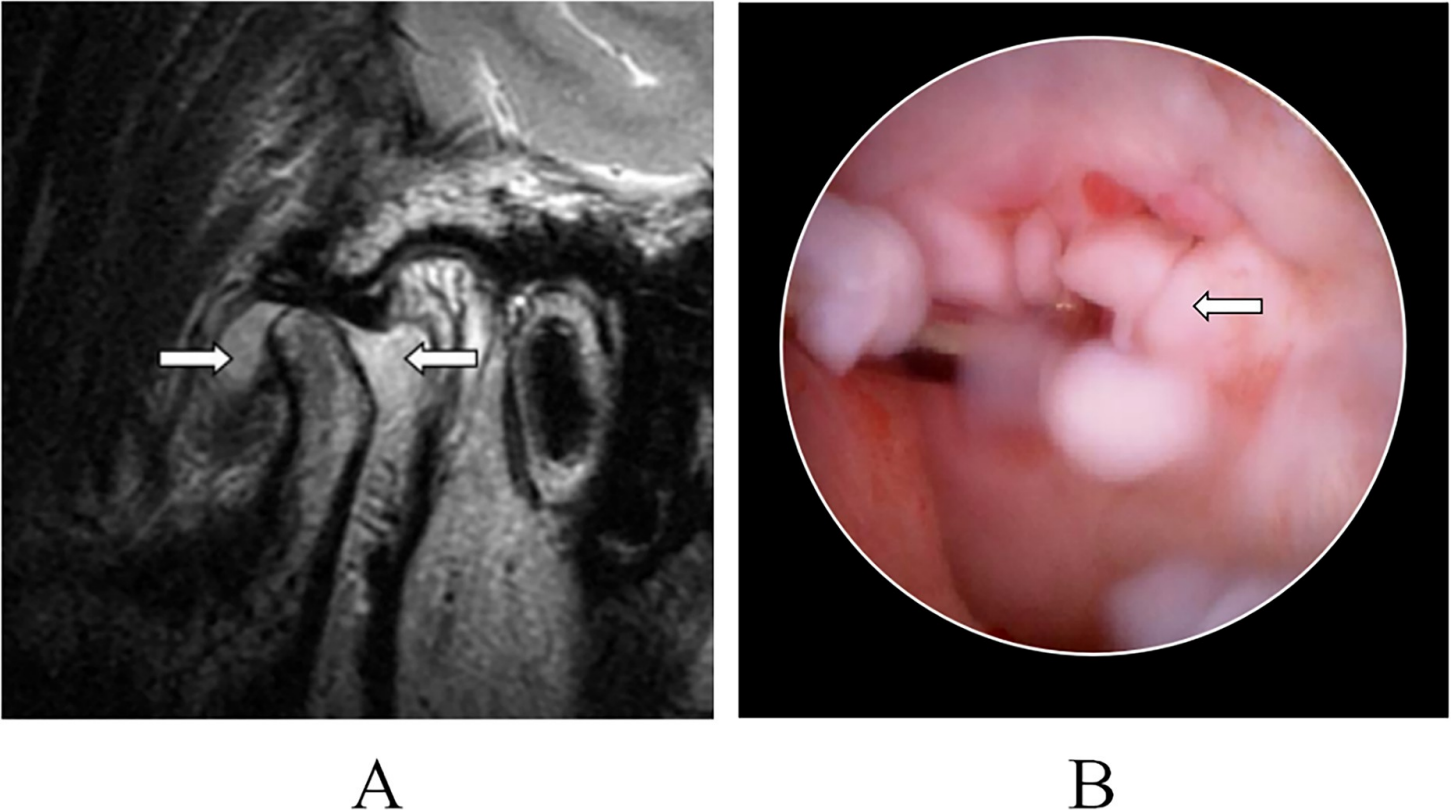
Synovial chondromatosis of temporomandibular joint: Hypointense loose bodies surrounded by hyperintense joint fluid (arrow) was detected on a T2 sequence of MRI, A; Isolated chondrified loose bodies (arrow) was detected during arthroscopic surgery, B.
